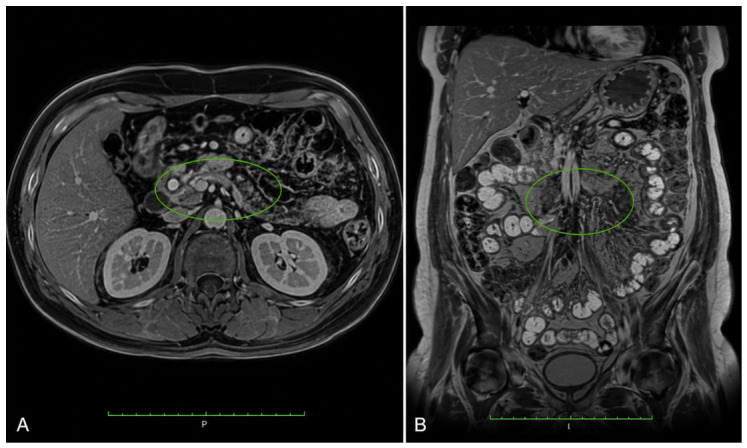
Mesenteric panniculitis—follow-up MRI at one month. (A) Axial opposed-phase T1-weighted image demonstrating complete resolution of the previously observed mesenteric fat infiltration, with no residual inflammatory lymph nodes at the mesenteric root (green oval). (B) Coronal T2-weighted fat-saturated image confirming complete resolution of the mesenteric fat infiltration and absence of residual inflammatory lymph nodes (green oval), consistent with complete radiological remission.
